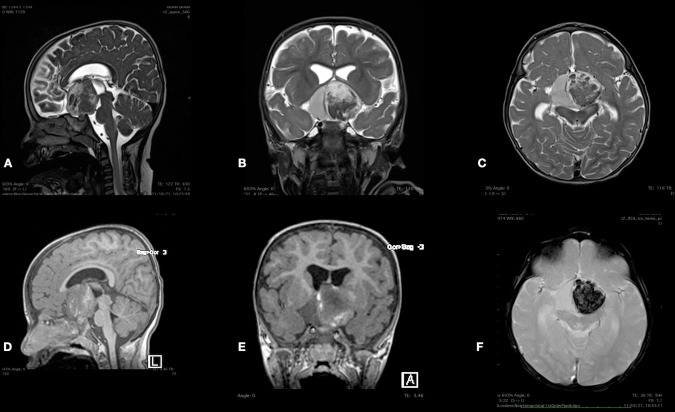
Sagittal (A), axial (B), and coronal (C) T2-WI and sagittal (D), axial (E) T1-WI MRI scan sequences of the brain demonstrating a large suprasellar mass with mixed solid and cystic components representing subacute hemorrhages. The T2-GRE sequence (F) revealed marked hypointensity of the tumor representing acute hemorrhage.
